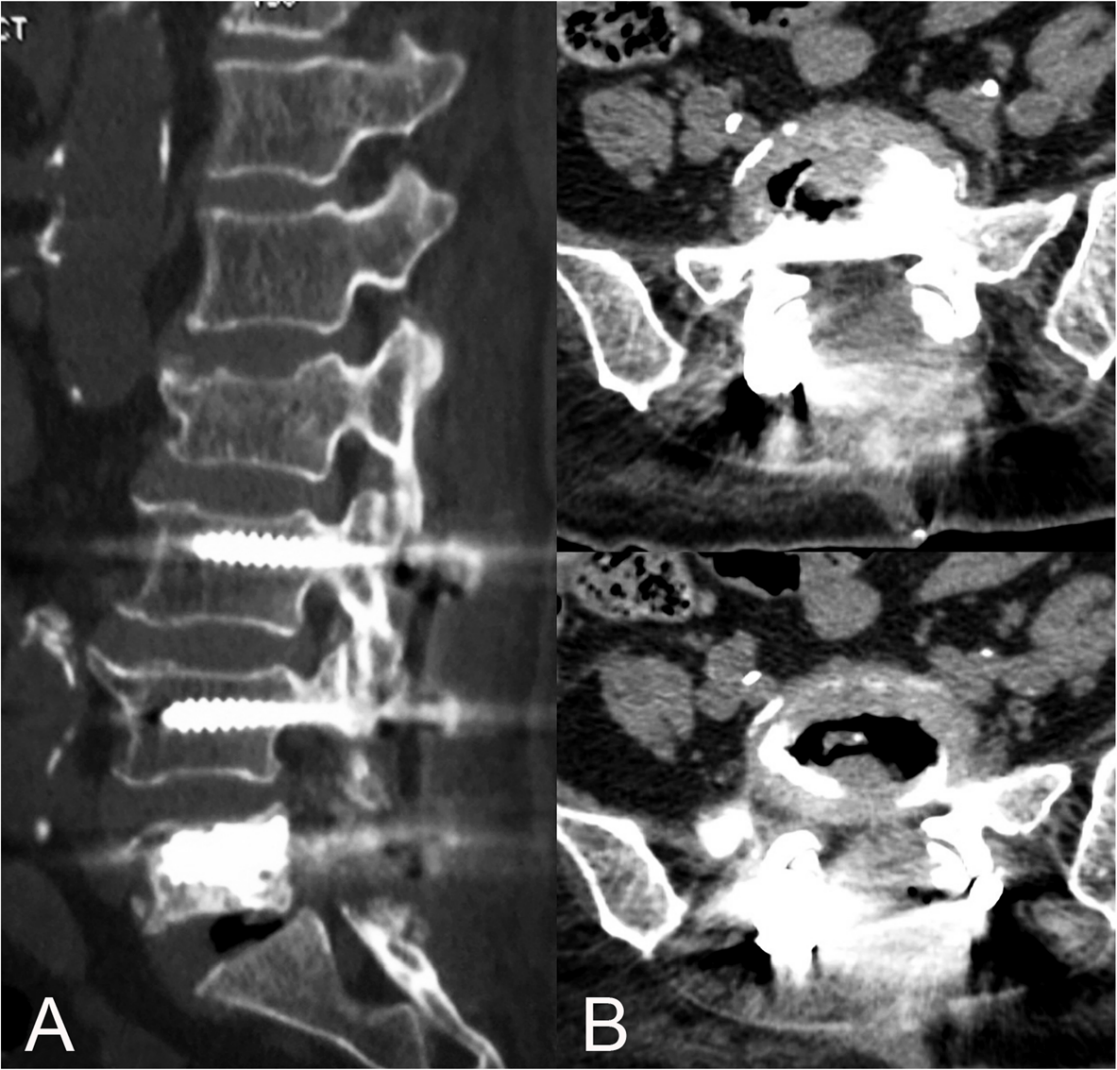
Postoperative sagittal (a) and axial (b) CT images demonstrated good alignment of the implant and the complete removal of epidural leaked cement with thorough decompression of the spinal cord at L5-S1
